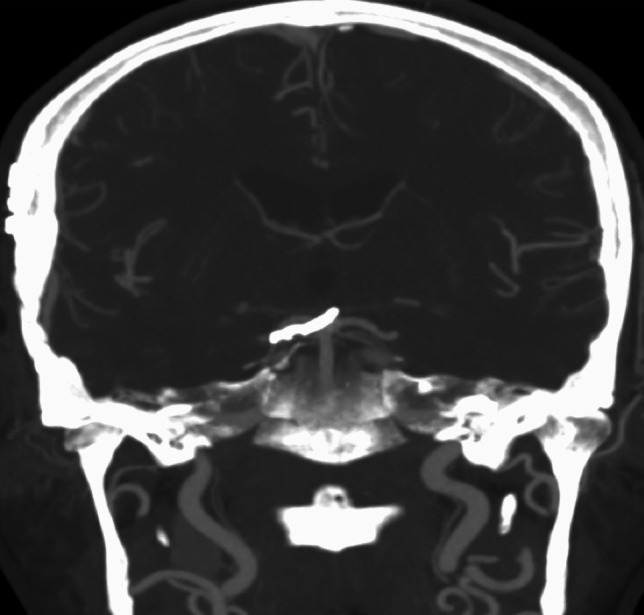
Postoperative CT angiogram showing complete clip occlusion of the basilar tip aneurysm
